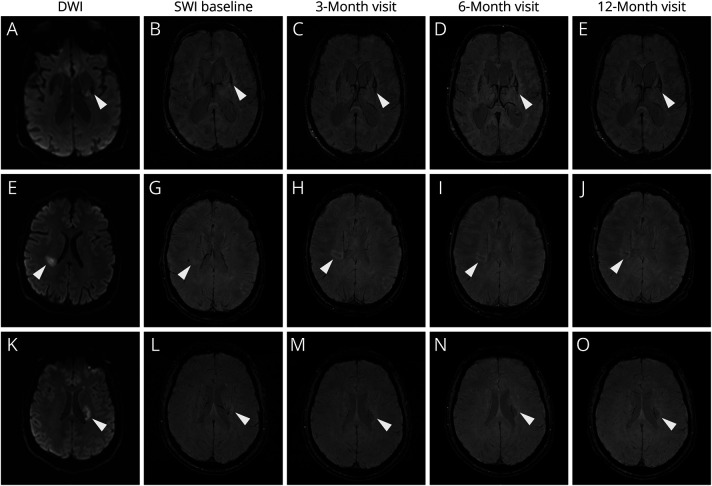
Morphology and Evolution of Hemosiderin Deposits (A–E) In a patient with an infarct in the basal ganglia, the “rim” pattern developed and became more obvious and thicker along the follow-ups. (F–J) In a patient with a RSSI adjacent to the lateral ventricle, the “smudge” became larger at 3-month and 6-month visits but shrank and turned darker, “condensed,” at the 12-month visit. (K–O) A “cluster” pattern in another RSSI adjacent to the lateral ventricle and the number of dots and lines became more visible inside and around the infarct during follow-up. DWI = diffusion-weighted imaging; RSSI = recent small subcortical infarct.
